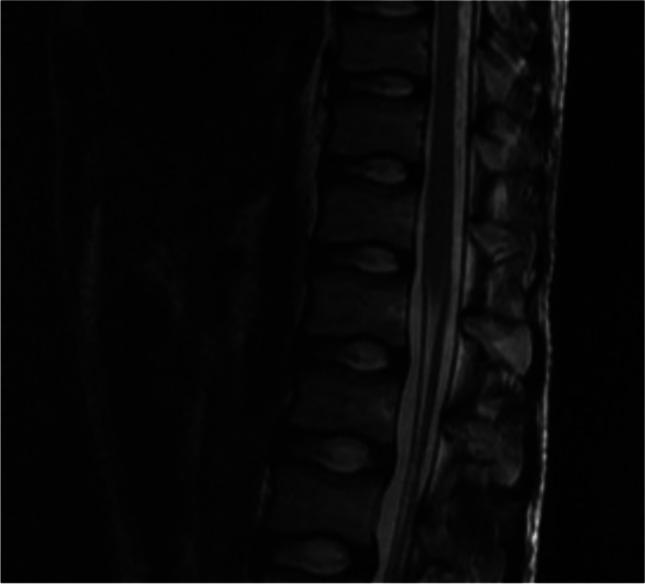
First RMN showing leptomeningeal thickening in T2-weighted image
